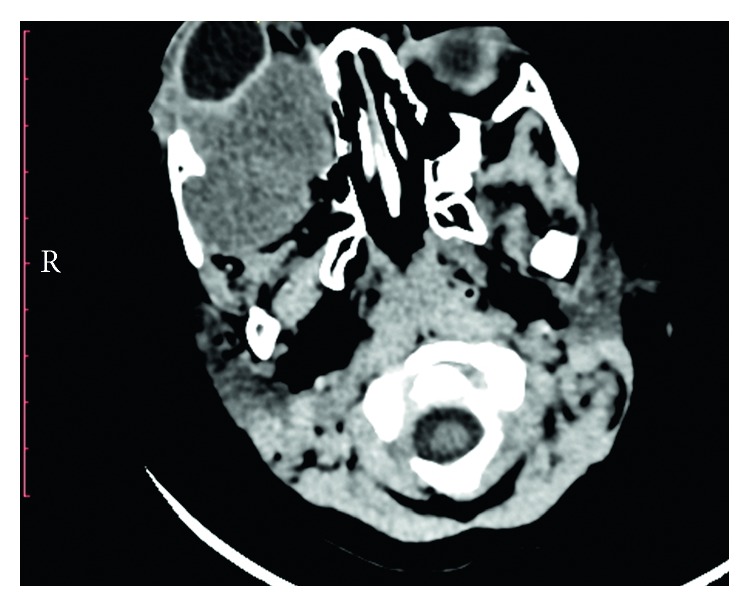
CT scan showing the neoplasm extended into the maxillary sinus and the temporal and infratemporal fossa.
